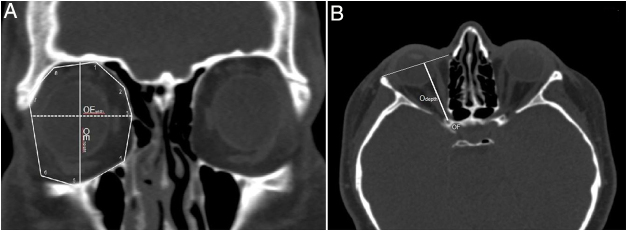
Multiplanar reconstruction of the CT images showing the morphometric measurements regarding orbital entrance and orbital depth. (A) The orbital rim landmarks were identified on octagonal polygon shaped orbital entrance in coronal plane: 1 supraorbital foramen, 3 frontoethmoidal suture and 2 midpoint between them, 4 ethmoidomaxillary suture, 5 infraorbital foramen, 7 zygomaticofrontal suture and 6 midpoint between them, 8 midpoint between zygomaticofrontal suture and supraorbital foramen. The area of this octagonal polygon was measured as orbital entrance area. Orbital entrance height (OE_height_) from infraorbital foramen to midpoint of the superior orbital rim and orbital entrance width (OE_width_) from frontoethmoidal suture to midpoint of the lateral orbital rim. (B) Orbital depth was taken as horizontal measurement from orbital foramen (OF) to orbital entrance.
